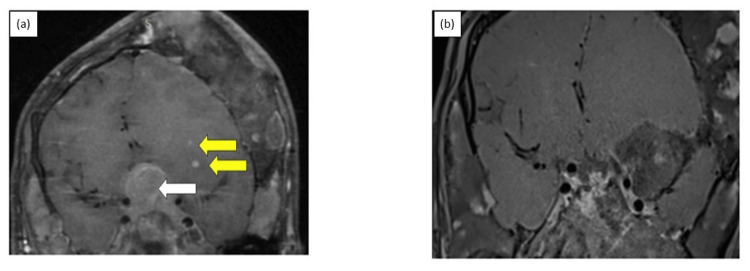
Preoperative post-contrast T1-weighted MRI sella and brain suggestive of (a) pituitary macroadenoma (white arrow) with multiple ring-enhancing lesions (yellow arrows) with (b) resolution of ring-enhancing lesion post-antitubercular therapy and decrease in the size of adenoma post-transsphenoidal surgery.
